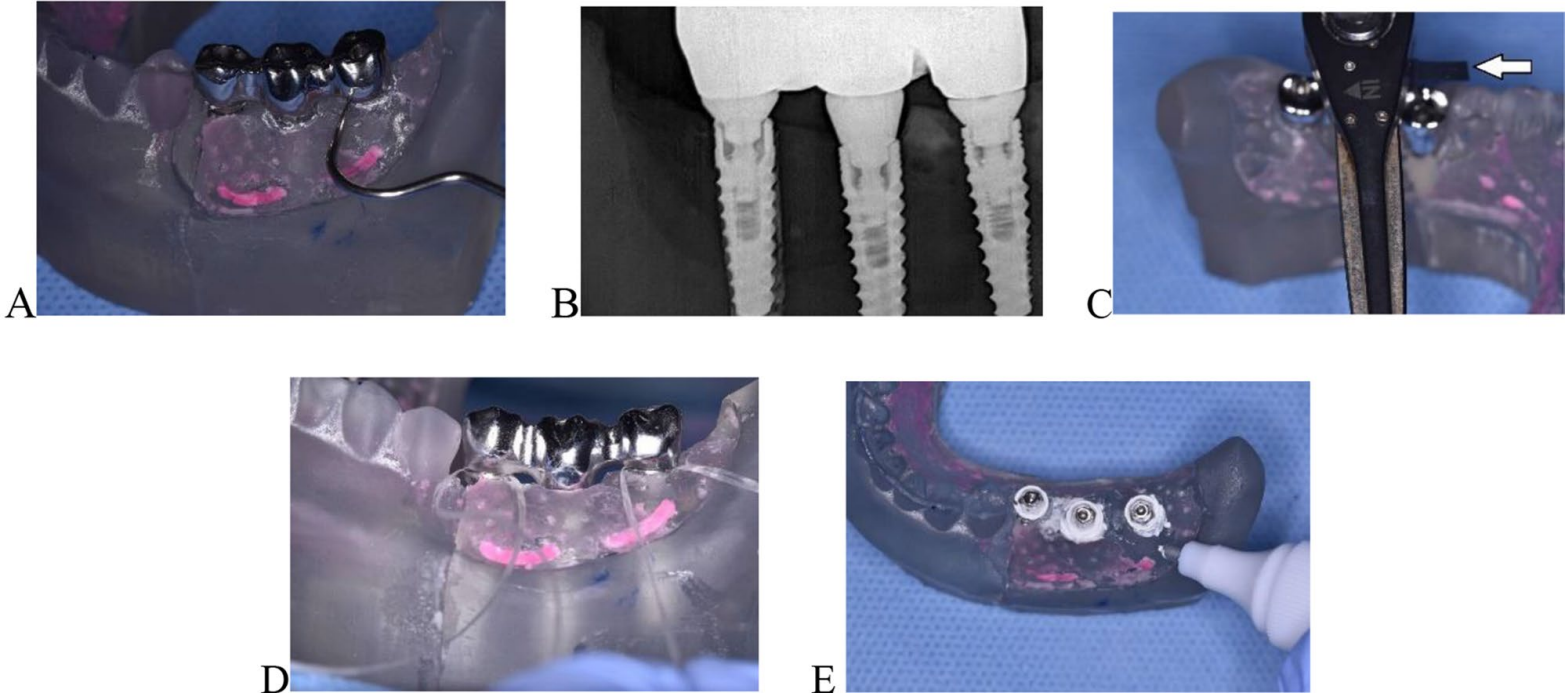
A, Tip of probe used for detecting gaps > 60 microns. B, Digital peri-apical radiograph to examine gaps between multi-unit abutments and framework. C, Flag test done by attaching a plumber’s tape on screwdriver, while pointing to central groove of first premolar and indicating if more than half turn was necessary to achieve passive fit. D, Floss slippage test to confirm findings of screw resistance test. E, Corrector pen used for checking fit of framework to abutments
